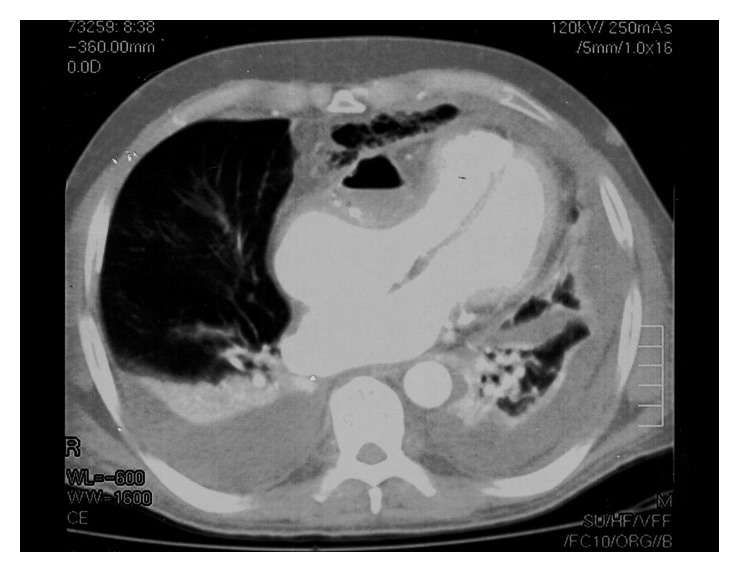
Chest CT scan. The mass is compressing the right ventricle and shifting the heart. Pleural effusion is present, with compression of the left lung.
